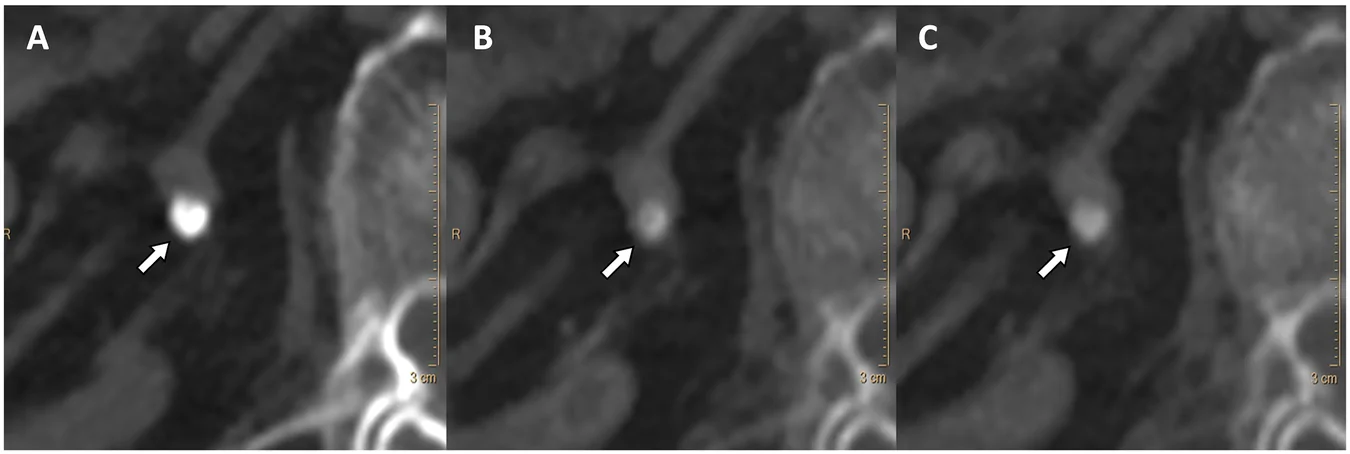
Axial CT images of right renal artery calcification in a 65-year-old male patient (arrow): A TNC with average calcification HU values of 885 ± 56, B VNC_A image, with average calcification HU values of 284 ± 30, C VNC_P image, with average calcification HU values of 310 ± 6. The calcification is less conspicuous in the VNC_A image (W 220 L 900)
